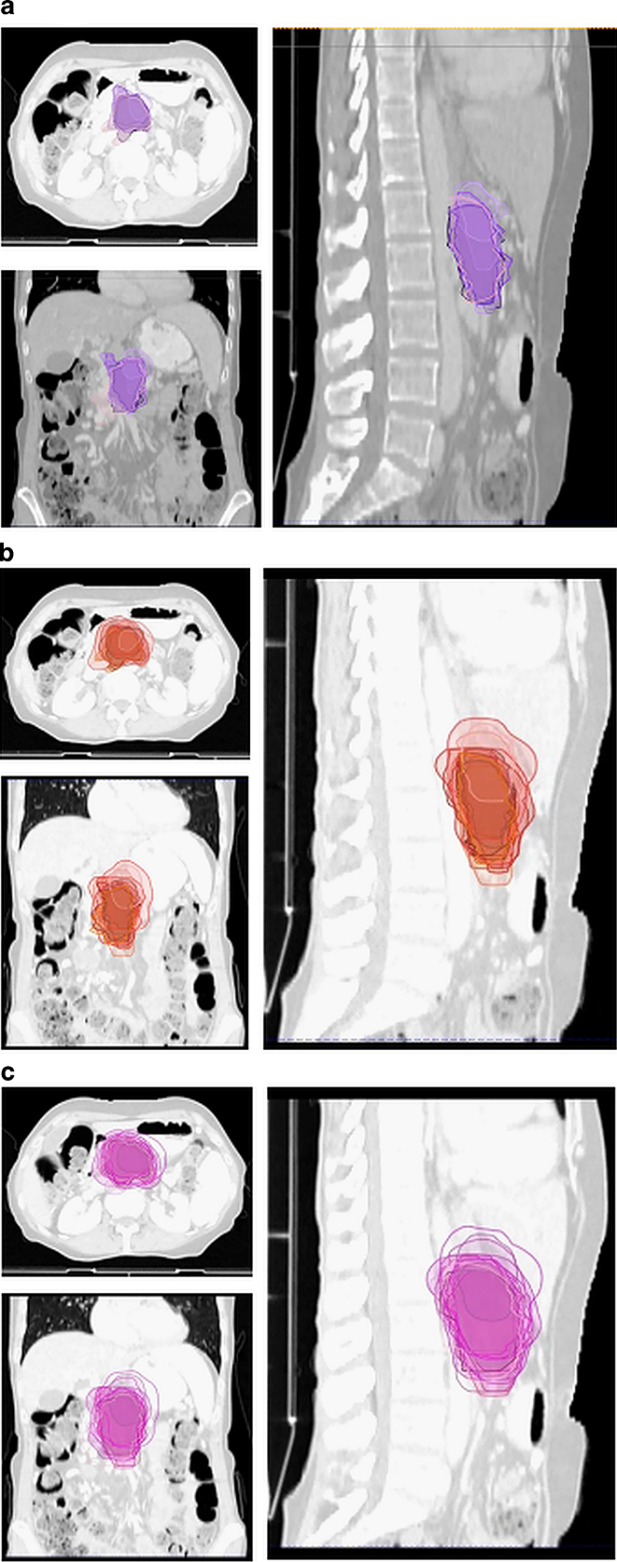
Case 3: patient with progression after initial chemotherapy. Definition of the target volumes performed based on 4D CT with IV contrast in combination with a diagnostic CT with IV contrast. a GTV, b ITV, c PTV
